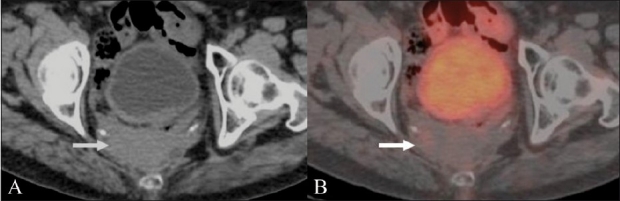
A 48-year-old woman with rectal cancer treated with APR and radiation therapy underwent a restaging FDG PET / CT for suspected recurrence. Axial unenhanced CT scan (A) shows a soft tissue mass in the presacral region (arrow). Fusion PET / CT image (B) shows no FDG concentration within the soft tissue (arrow), suggesting post-treatment fibrosis
